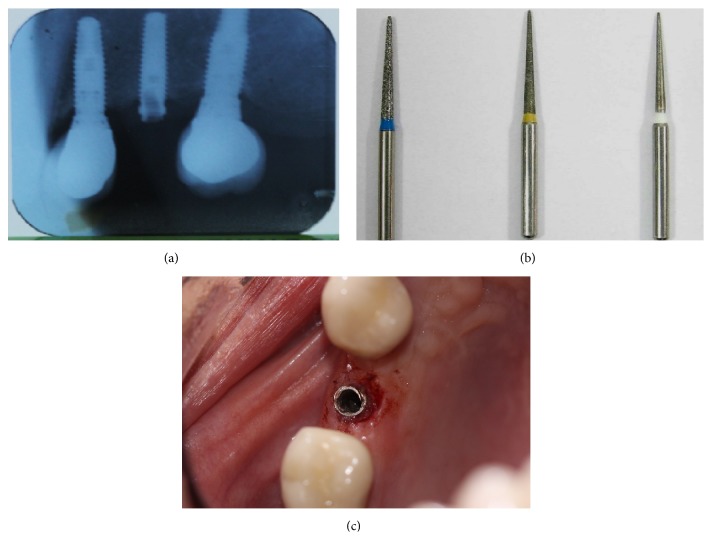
Removal of fracture fragment: (a) radiograph; (b) fissure bur was used to remove the fractured screw; (c) occlusal view.
